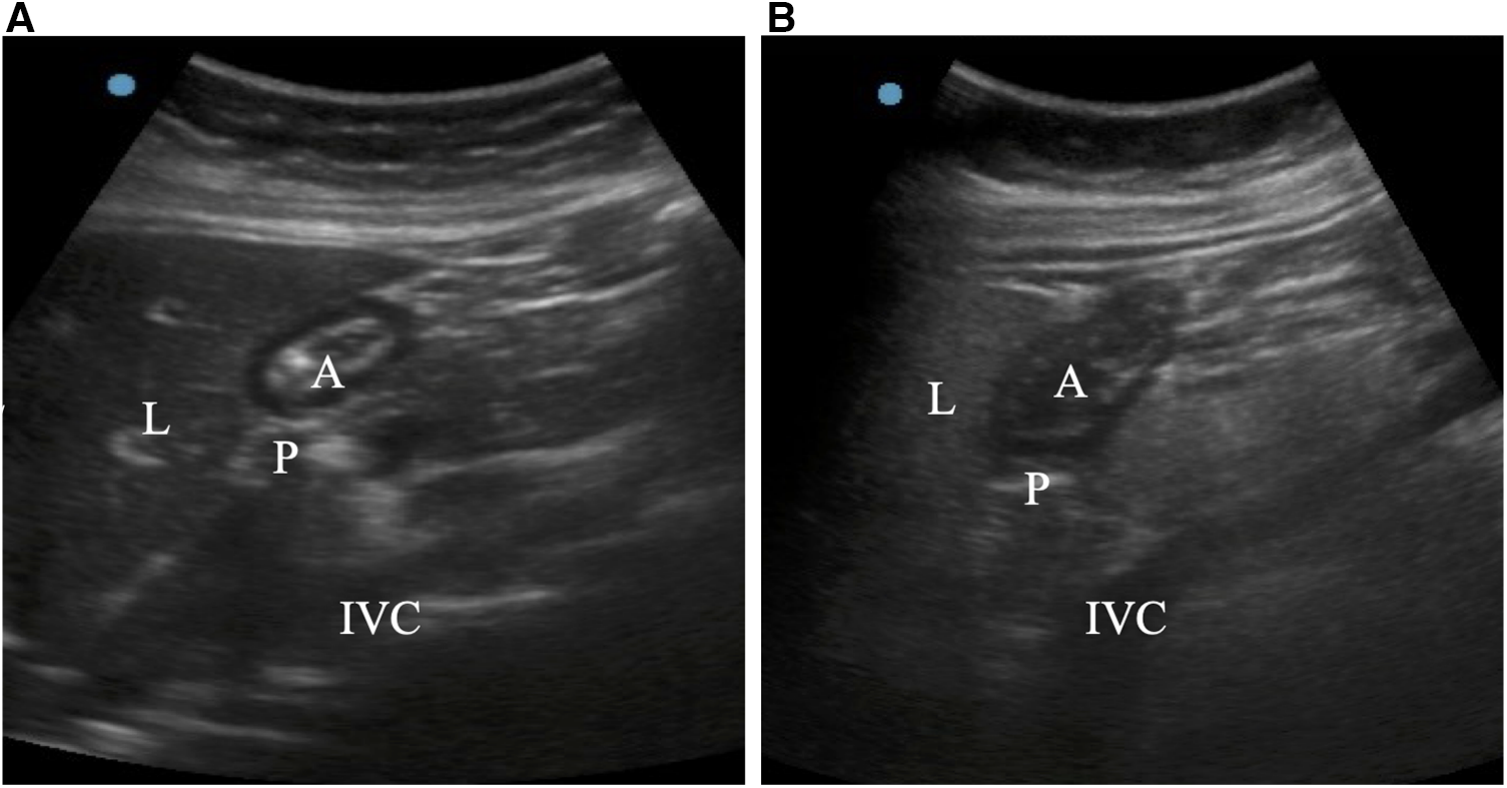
Ultrasound images of the stomach antrum when it was in the right lateral decubitus position. In an empty stomach, the gastric antrum appears flat and folded (A). If fluid content is present, the stomach antrum appears swollen and hypoechoic (B). A = antrum; IVC = inferior vena cava; L = liver; P = pancreas.
